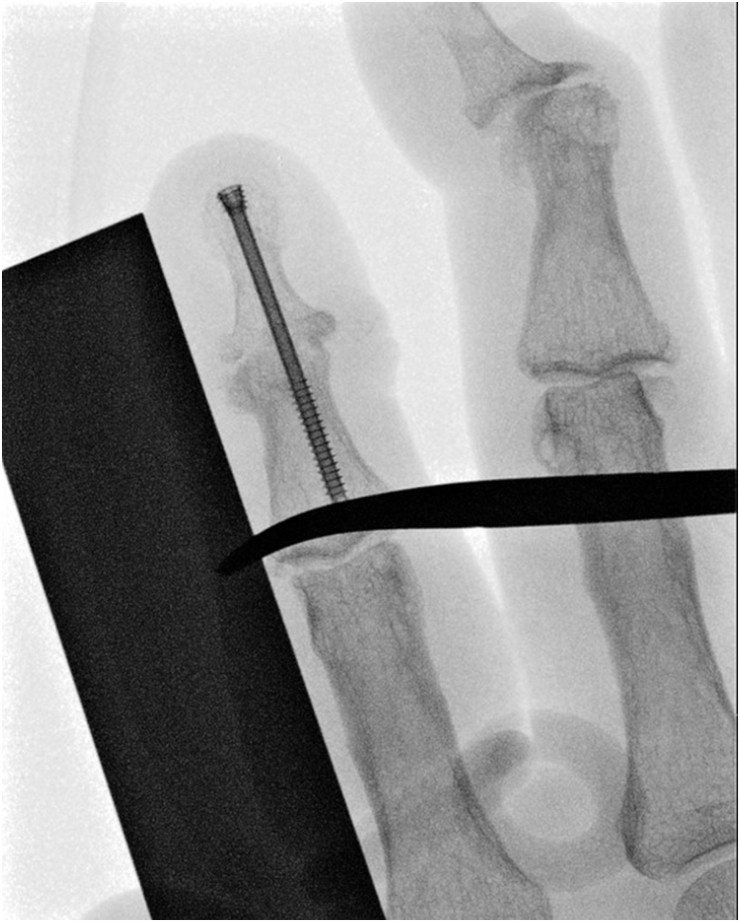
X-ray image of headless compression screw accurately positioned through the distal interphalangeal joint of the right index finger.
